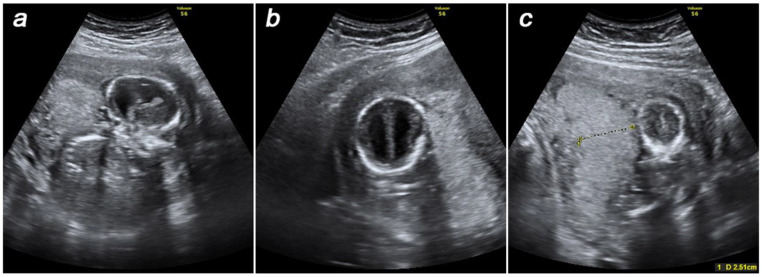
Prenatal ultrasound findings at 16–17 weeks of gestation: dolichocephaly (a), ventriculomegaly (b), thick placenta (c).
